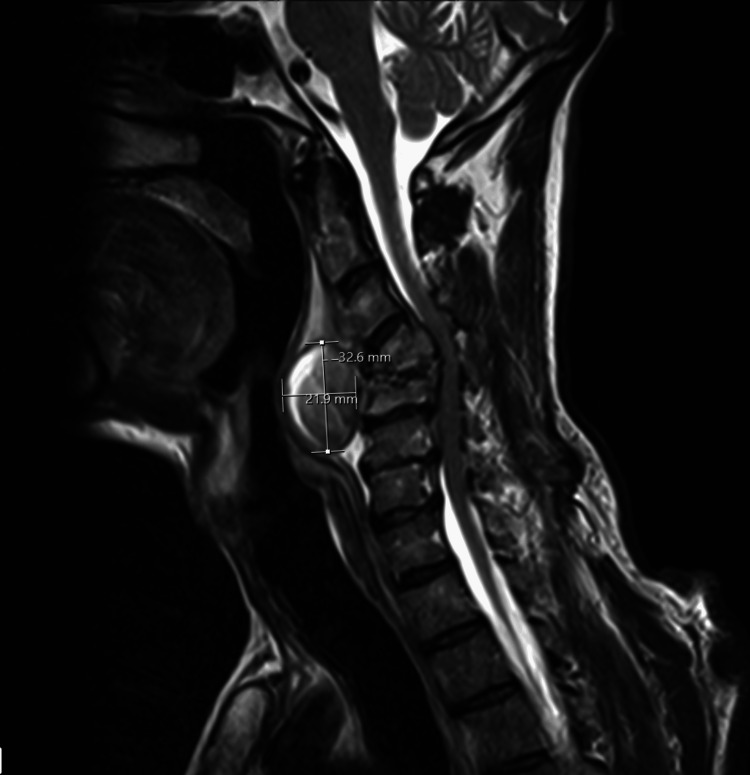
MRI spine with contrast with T2 sequence, done at the time of admission admission. There is a rim enhancing multiloculated collection measuring at least 5.5x3.5x2.5 cm in the prevertebral soft tissues in continuity with the C4-5 disc
